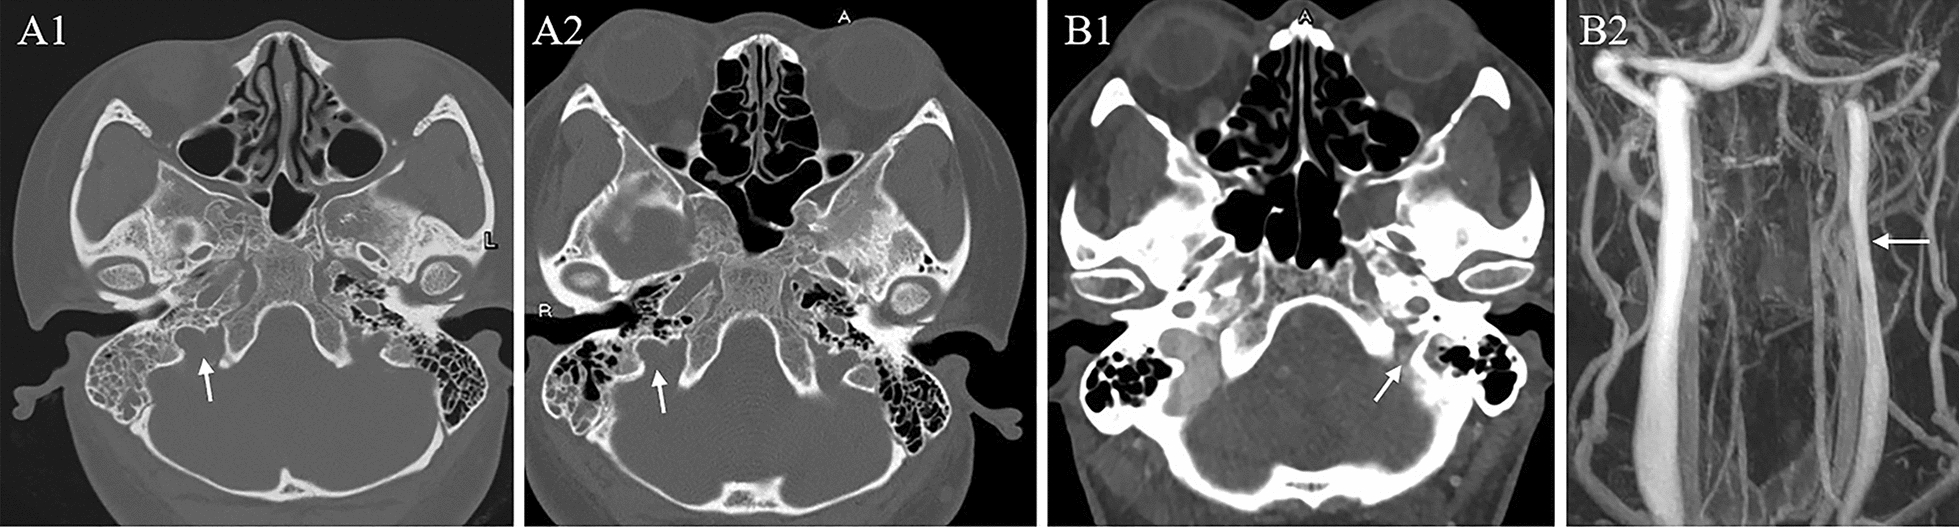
Initial and follow-up computed tomography (CT) maps, and a representative case with jugular hypoplasia. Right jugular foramen (JF, white thin arrow) on baseline CT image (A1) appeared to be the same as that on 2-year CT follow-up (A2). A 39-year-old male for physical screening was imaged with narrowed ipsilateral JF (B1, white thin arrow) along with hypoplastic IJV (B2, white thin arrow) on CE-MRV
